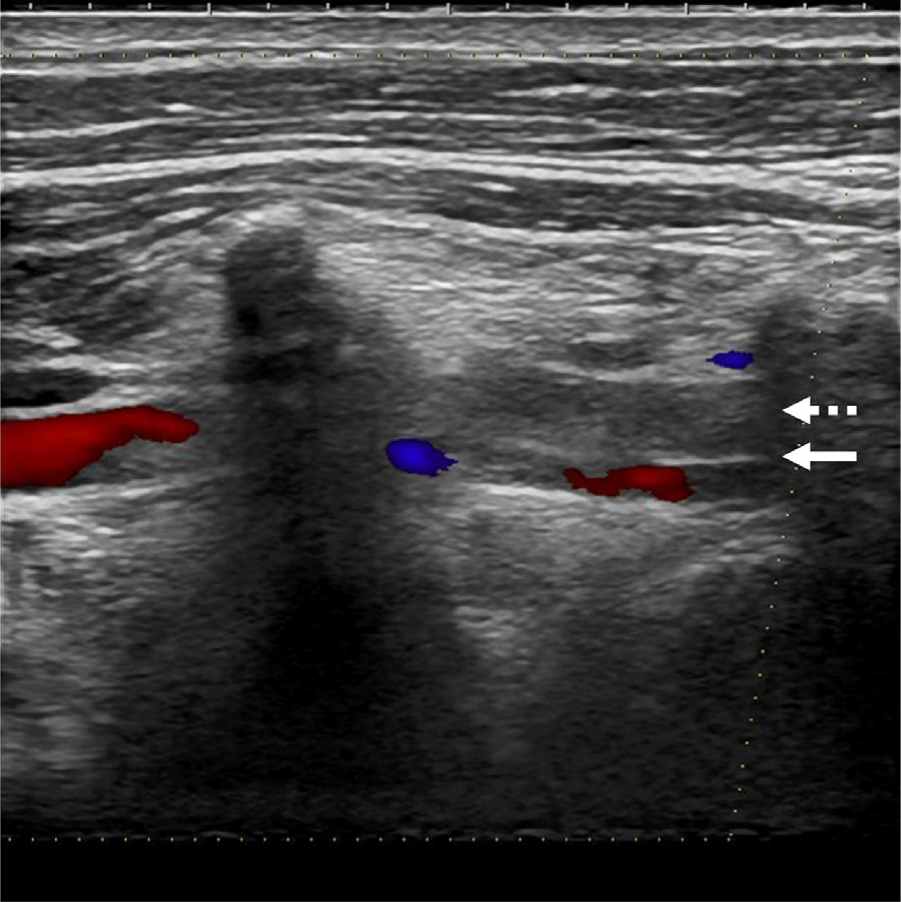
Extracranial vertebral artery dissection diagnosed by ultrasonography. Ultrasonography demonstrating an intimal flap (arrow) and decreased blood flow signal within the right extracranial vertebral artery. The dotted arrow indicates an intramural hematoma.
